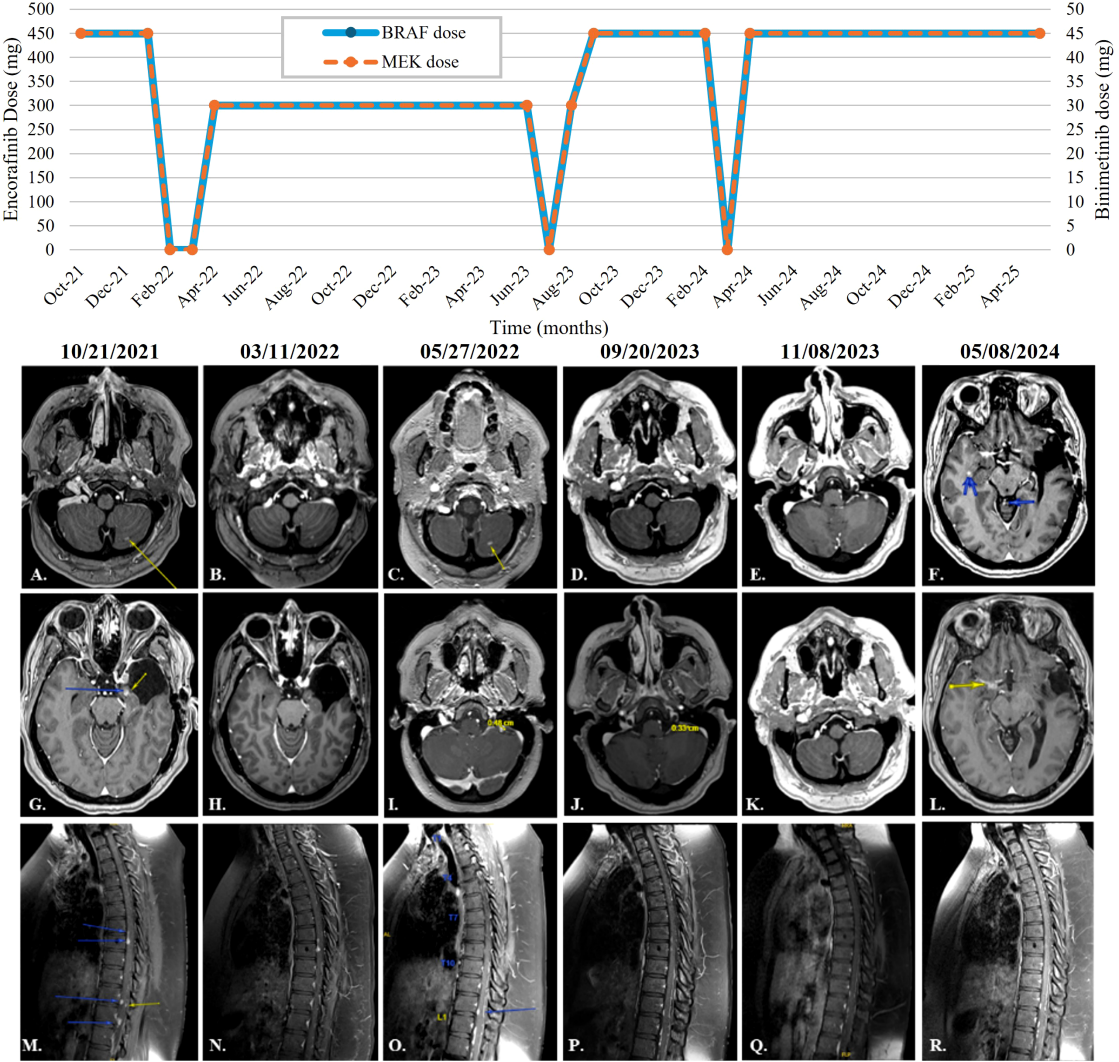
BRAF / MEK inhibitors dose through time and patient response. (A, G, M) show patient's baseline at time of LMD diagnosis and starting BRAF/MEKi therapy (B, H, N) show progression after therapy was held for four weeks. (C, I, O) show radiographic control and size reduction of enhancing nodular areas. (D, J, P) show new areas of enhancement after three days withholding the reduction dose of BRAF/MEKi. (E, K, Q) show radiographic improvement again after restarting full dose of BRAF/MEKi. (F, L, R) show new nodular enhancement in several areas of the CNS including a new temporal lesion after 2 weeks withholding BRAF/MEKi.
